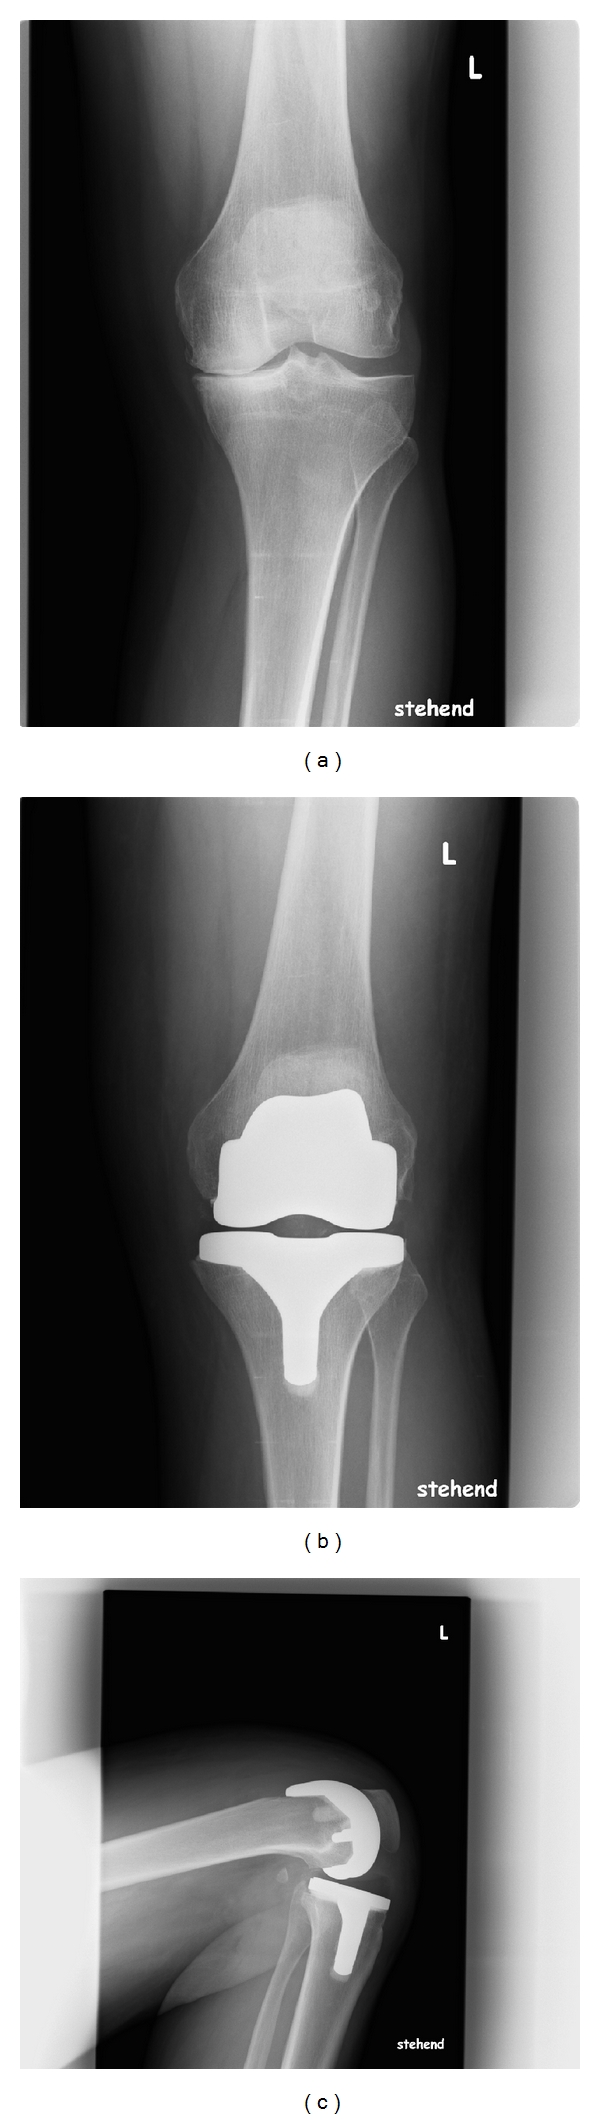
X-ray of a Columbus knee: a.p. view pre- and postoperatively (standing position with full weight bearing) and lateral view in maximum flexion. There is soft tissue contact of calf and tight. The reduced length of the posterior implant condyles led to bone contact of the remaining original posterior condyles with the posterior edge of the polyethylene inlay.
